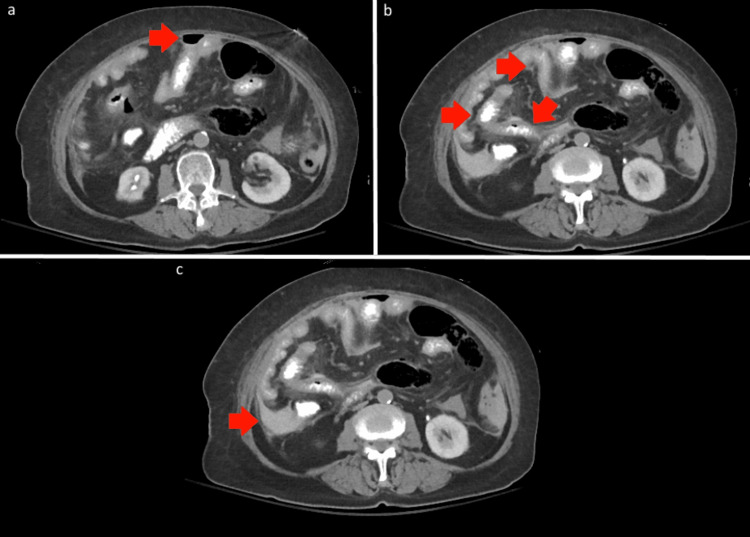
Computed tomography after initial patient presentation showing (a) intraperitoneal free air, (b) small bowel wall thickening, and (c) intraperitoneal fluid collection
